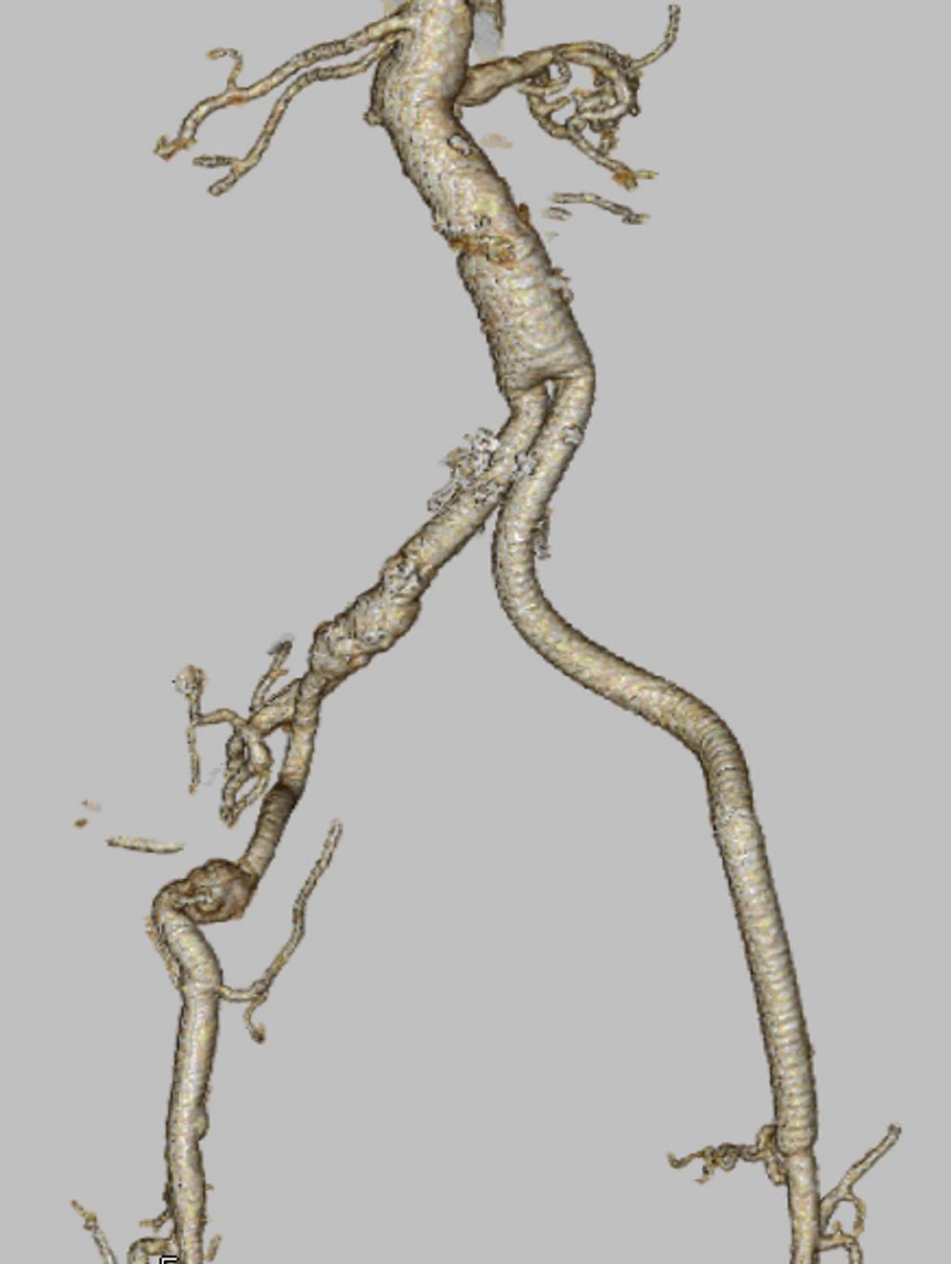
Postoperative computed tomography. 3D image showed good anastomosis and no aneurysm.
